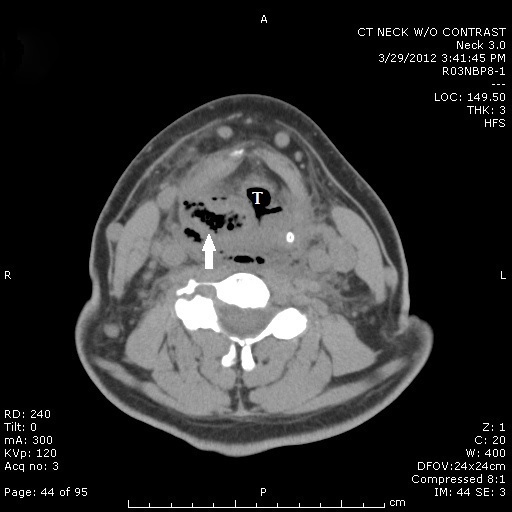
Computed tomography (CT) of the neck demonstrating soft tissue swelling, liquid and air collection within the oropharynx (arrow) near the trachea (labeled T).
